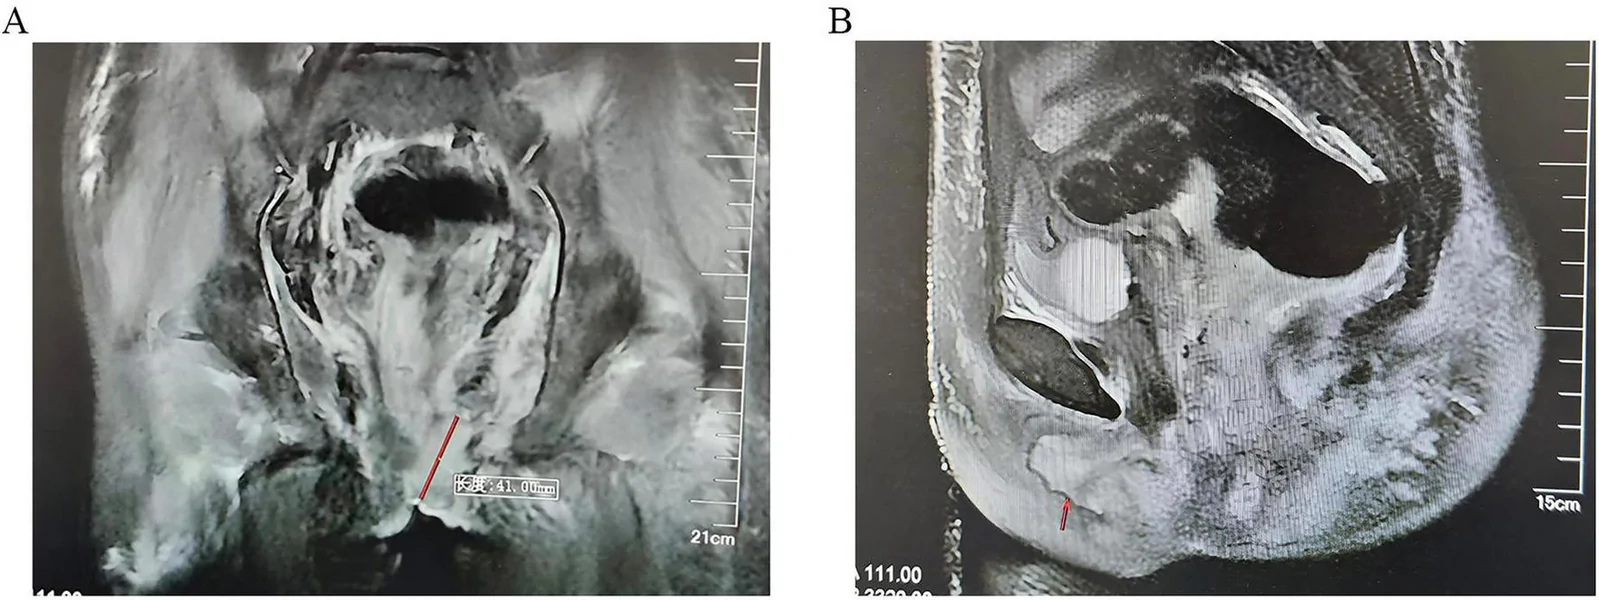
Enhanced magnetic resonance imaging of the vulva. (A) Coronal view (with red markings indicating the size of the lesion). (B) Sagittal view (with red arrows indicating the location of the lesion).
